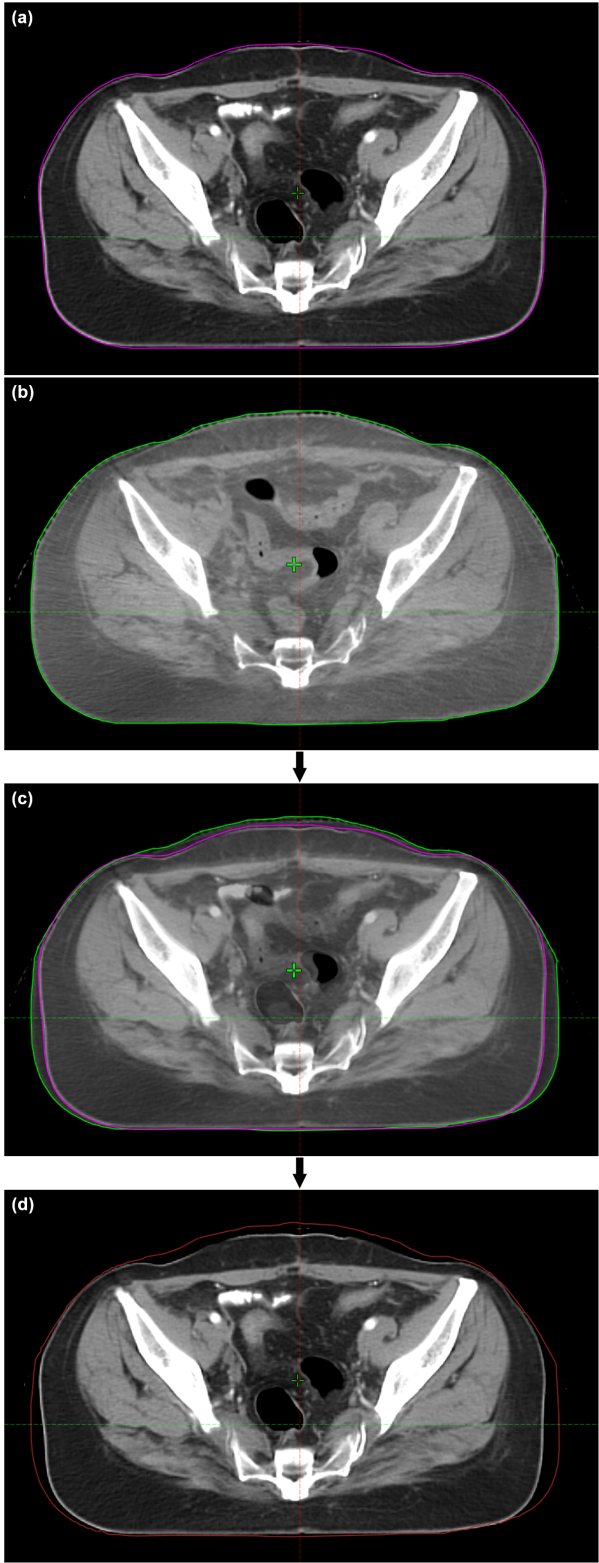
Demonstration of vCT generation for a patient. (A) pCT; (B) CBCT; (C) CBCT–pCT registration; (D) vCT. The CT values in the added area within the updated body contour of vCT are set as 0 HU.
